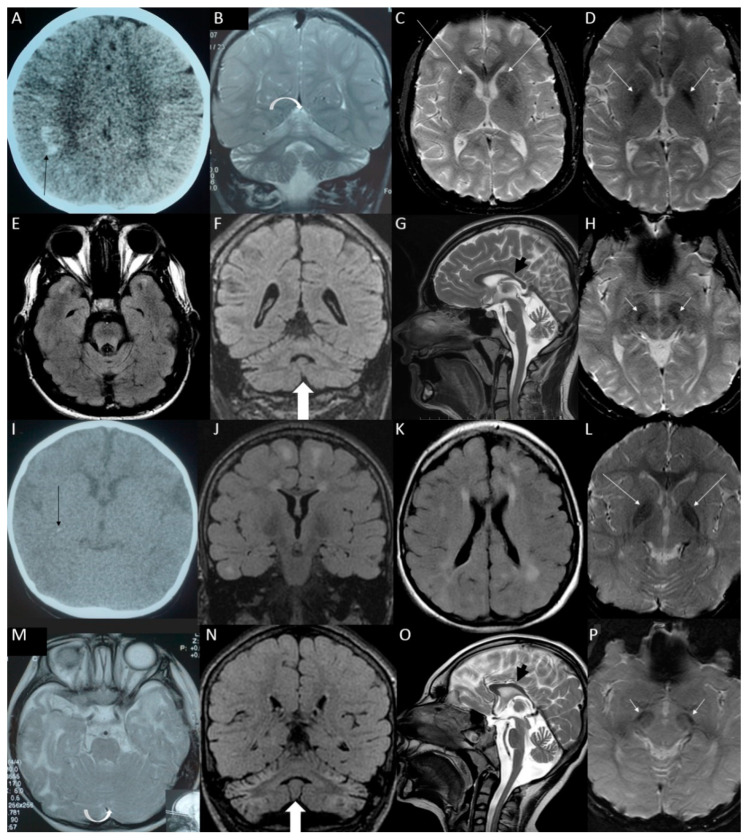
Brain imaging of sibling with *SUPV3L1* homozygous variant c.1093C>T:p.(Arg365Trp)—the older brother (patient S19a) in panels. (A) Computed tomography (CT) at the early childhood with marked subcortical calcifications in both frontal and parietal lobes (black arrow). (B) Subsequent magnetic resonance imaging (MRI) scans at the middle childhood with marked shrunken bright cerebellum (white arrow). (C–H) Brain MRI at the late adolescence. (E) FLAIR hyperintensities remained in the temporal poles only, (F) atrophic cerebellum but not so FLAIR-hyperintense as earlier and as in the younger sister (white arrow), (G) the corpus callosum is shorter and thinner than normal (black arrow), (D) iron or calcium deposits in the globi pallidi (white arrows), (H) in substantia nigra (white arrows) (C) and in the caudate nuclei (white arrows); brain imaging of sibling with *SUPV3L1* homozygous variant c.1093C>T:p.(Arg365Trp)—the younger sister (patient S19b): (I) CT at the early childhood revealed two punctate subcortical calcifications in the right cerebral hemisphere (black arrow). (M) MRI taken as the toddler with normal cerebellum with slight widening of the cerebellar sulci (white arrow). (J–P) MRI at the early adolescence: (J,K) myelination has progressed, but there are white matter hyperintensities on FLAIR sequence in both cerebral hemispheres, (N) shrunken bright cerebellum (white arrow), (O) shortened and thinned corpus callosum (black arrow), (L) iron or calcium deposits in the globi pallidi (white arrows) (P) and in substantia nigra (white arrows).
